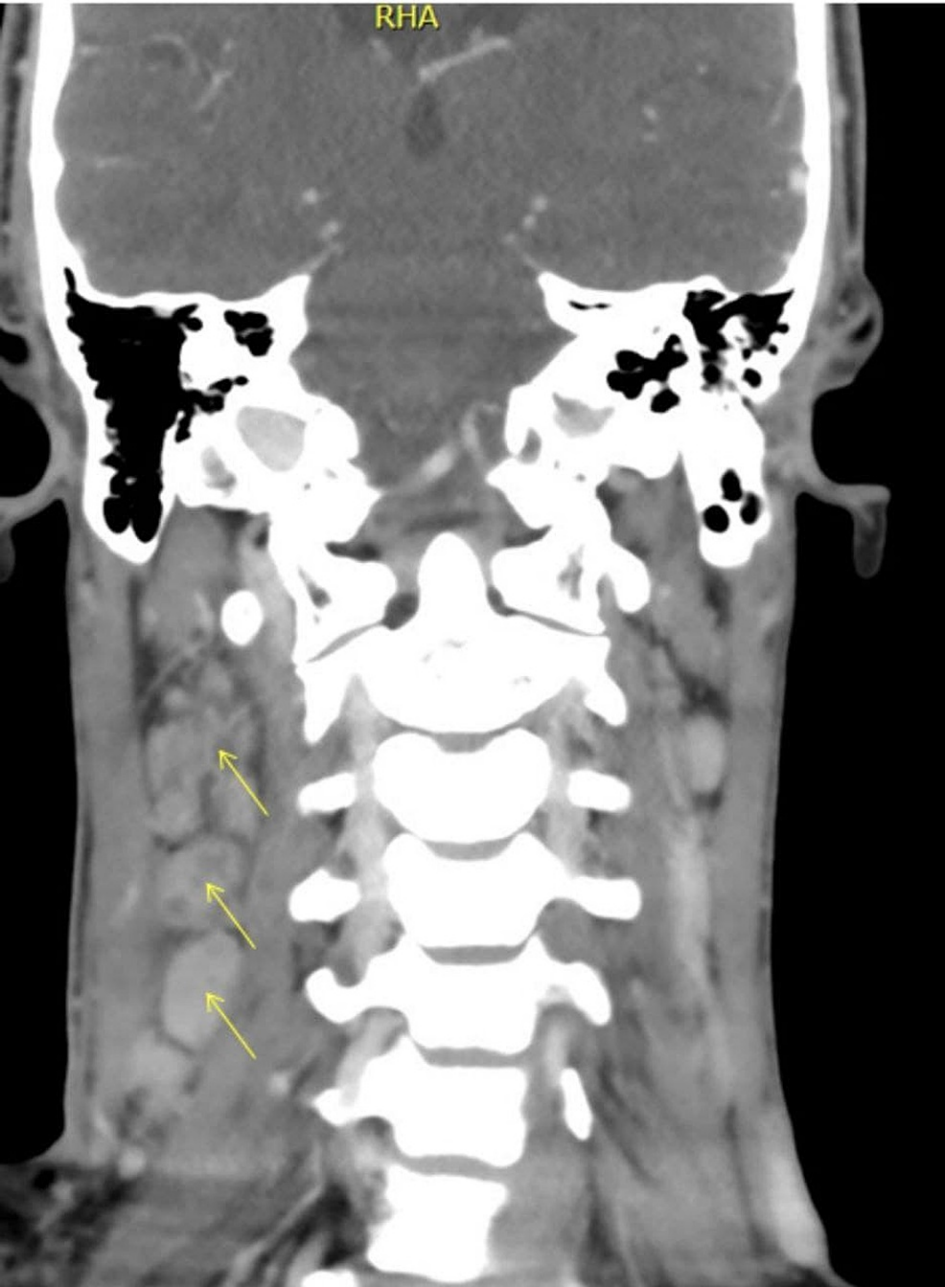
Neck CT: sagittal section of right deep cervical lymphadenopathy with central necrosis.
